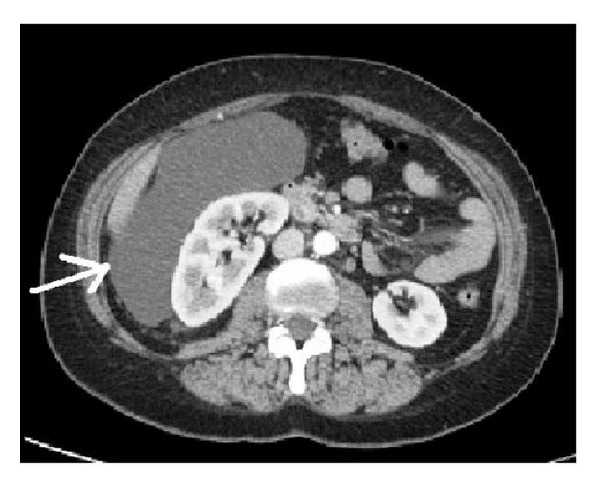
Computed tomography (CT) of the abdomen and the pelvis confirming the presence of a 22 × 19 × 8 cm retroperitoneal mass, extending anteriorly to abdominal wall of right upper quadrant and winding the entire distal right kidney.
